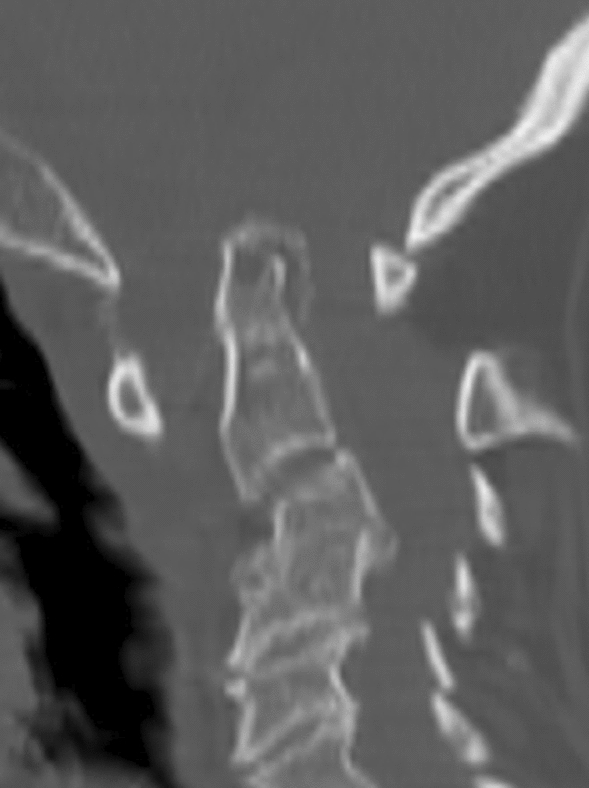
Sagittal CT reconstruction depicting several findings consistent with increased anterior atlantoaxial distance and cephalad migration of C2 (cranial settling), findings consistent with atlantoaxial subluxation and atlantoaxial impaction.
